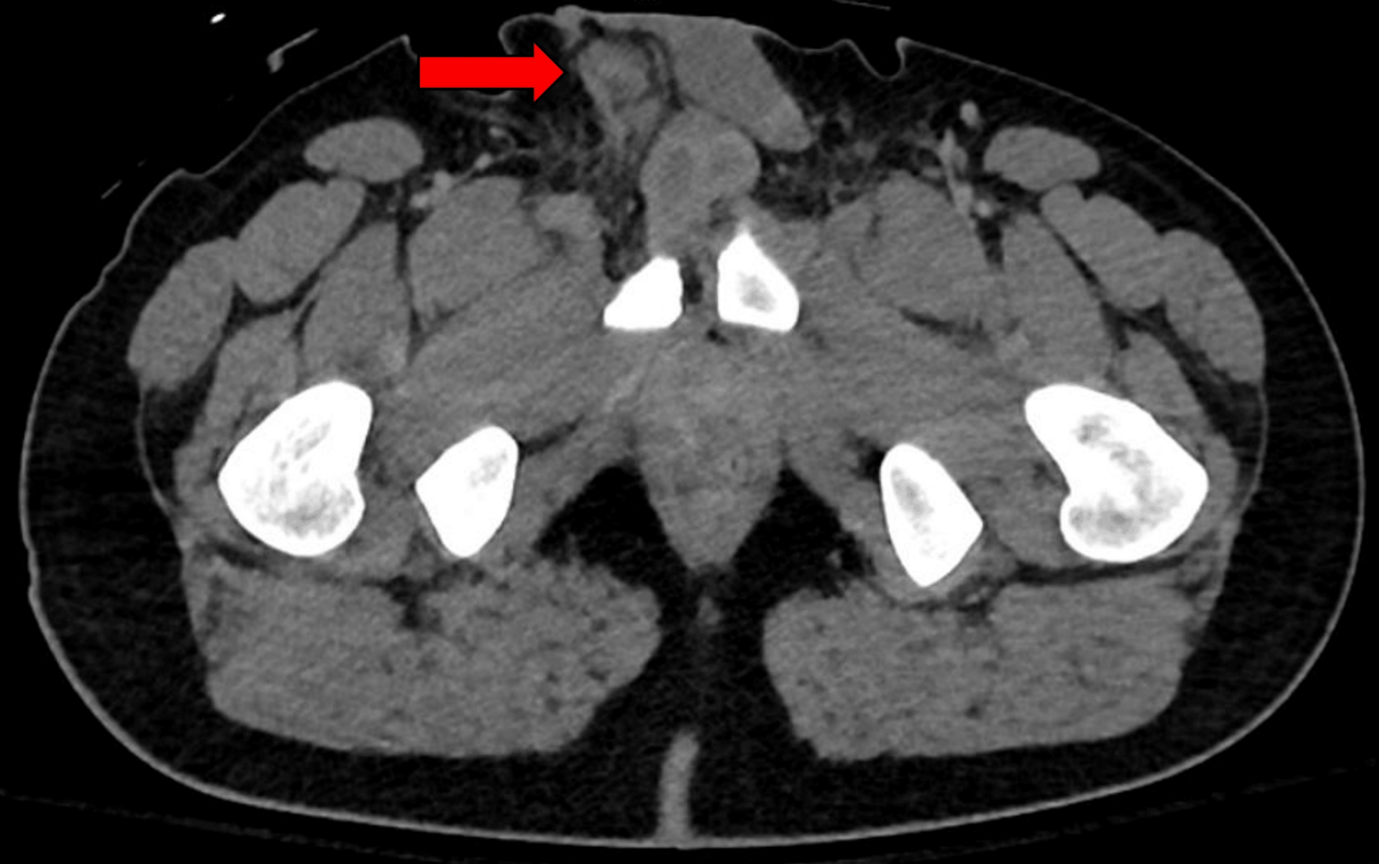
CT pelvis demonstrating an incidental finding of the right testis elevated in the inguinal canal (red arrow). CT: computed tomography
